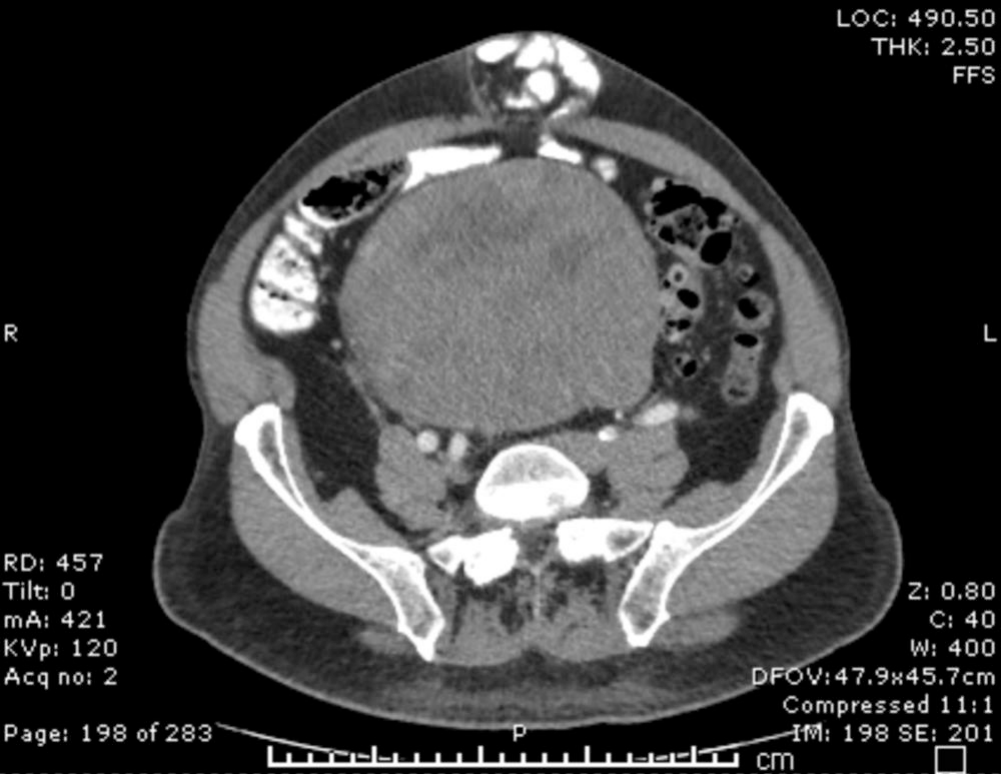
Computed tomography of abdomen and pelvis. Large mass in the lower abdomen and pelvis measuring 20.5 cm.
